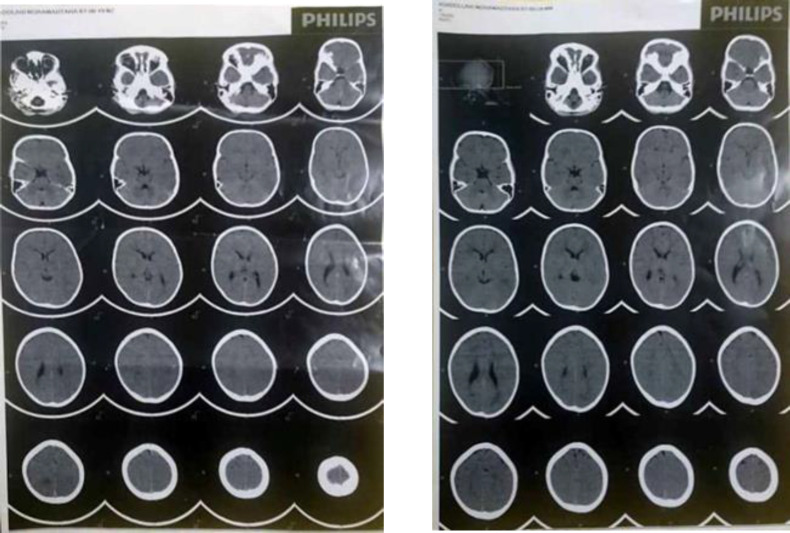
Brain MRI of three and half year old boy (signal change in external capsule, arrested hydrocephaly and hypomyelination in basal ganglia)
